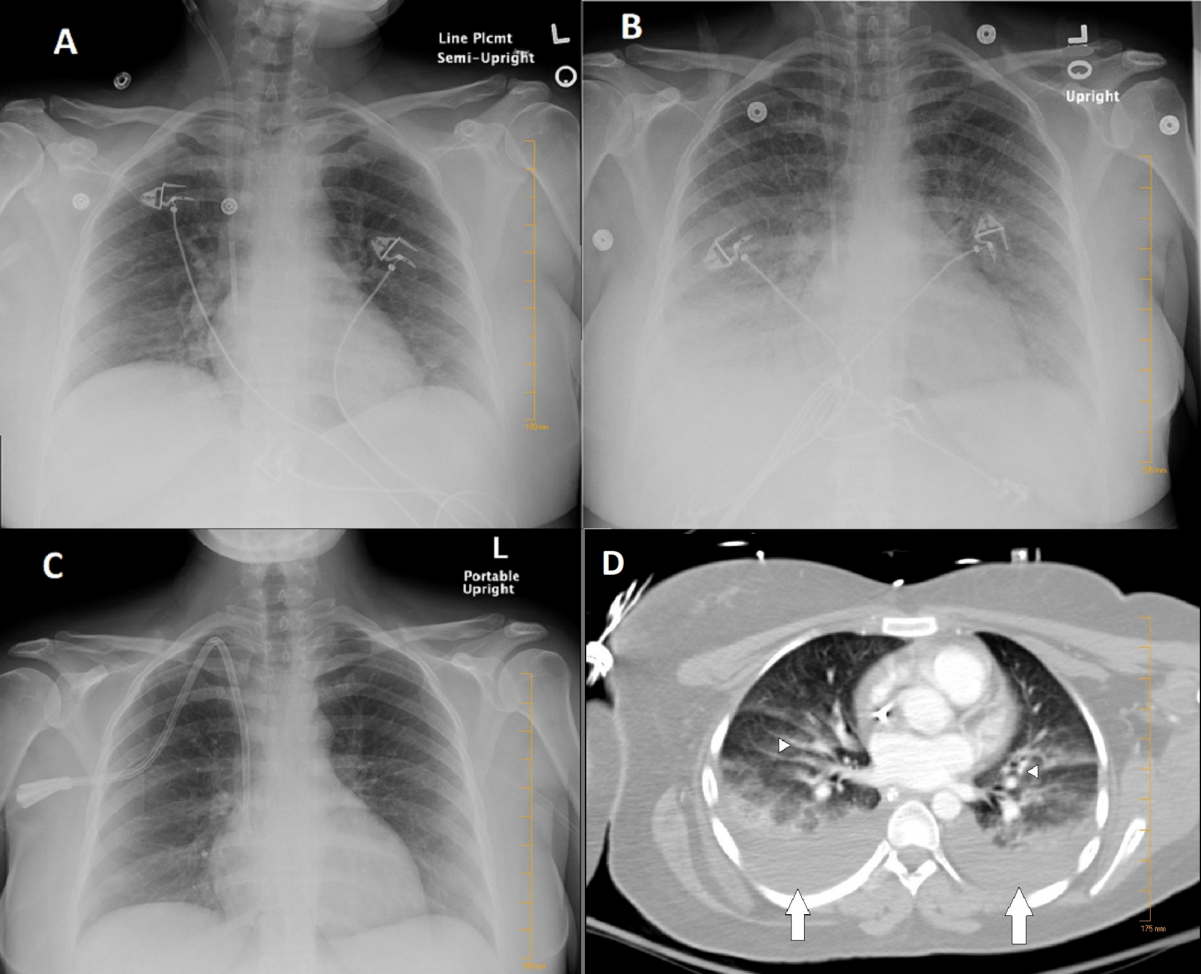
Chest radiographs and computed tomography (CT) of the patient during her hospital course A) No acute intrapulmonary process, normal cardiac size; B) bilateral perihilar interstitial opacities and vascular congestion suggestive of interstitial pulmonary edema; C) complete resolution of vascular congestion. The heart is normal in size and contour; pulmonary vessels are normal in size and caliber; D) contrast-enhanced computed tomography (CT) of the chest showing bilateral pleural effusion (white arrows) and diffuse bilateral vascular congestion (arrowheads) suggestive of pulmonary edema; no pulmonary embolism noted.
